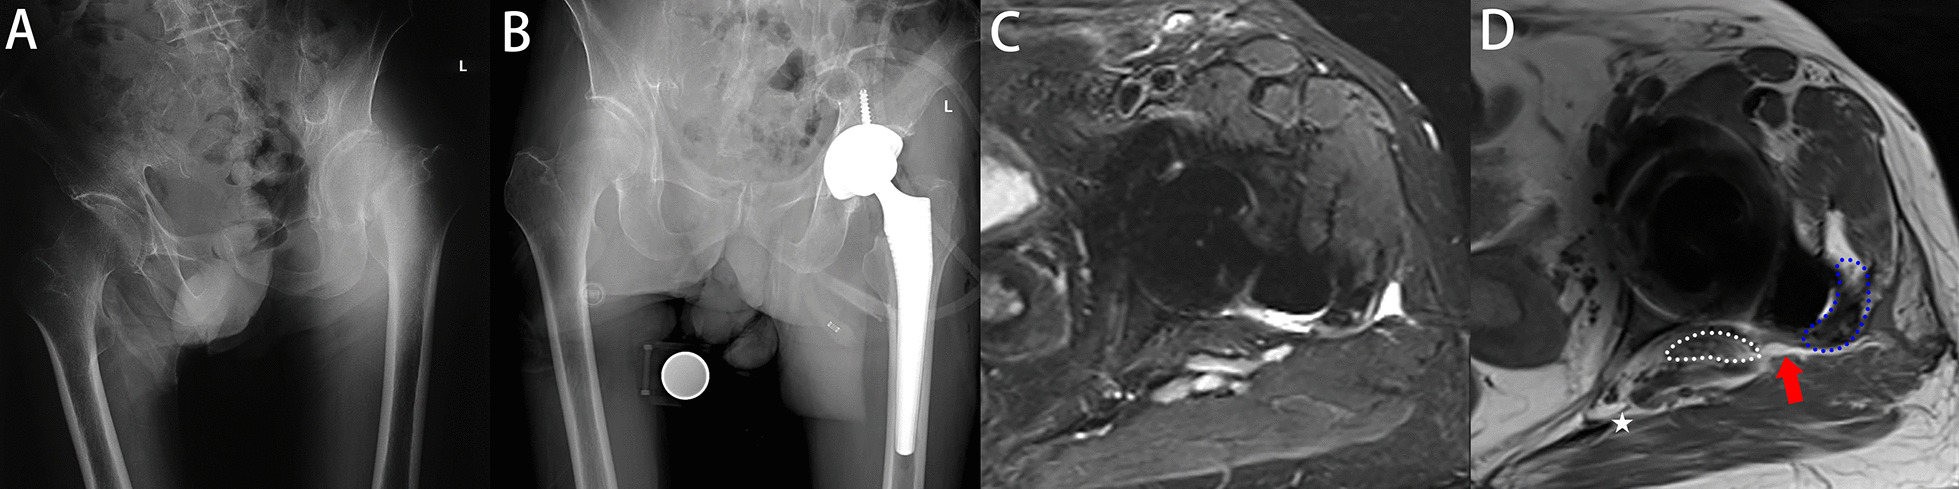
Female, 75 years old, femoral neck fracture (A). THA with mesh reconstruction was performed (B). Follow-up MRI (C, D) showed a gap between mesh and femur (red arrows). Symbols are identified as above
